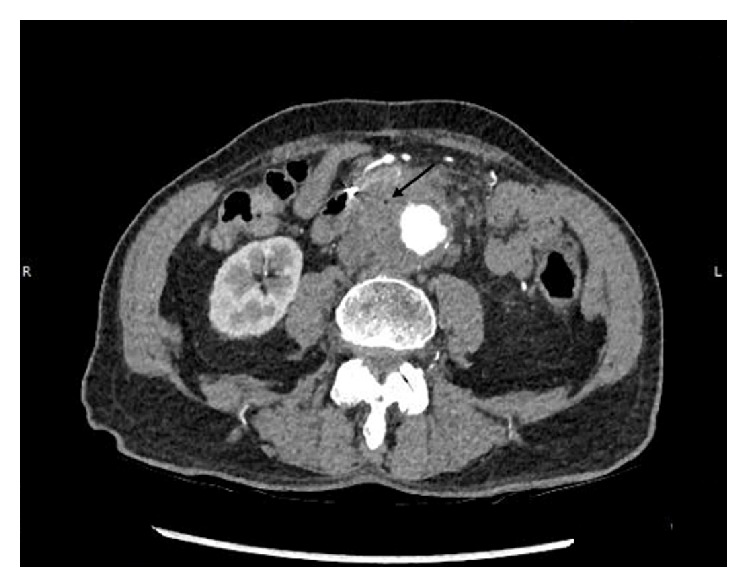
CTA showing a 5.3 cm aortic aneurysm, marked soft tissue thickening of the aortic wall, and a small pocket of gas within the soft tissue of the aorta (soft tissue gas designated by the arrow).
